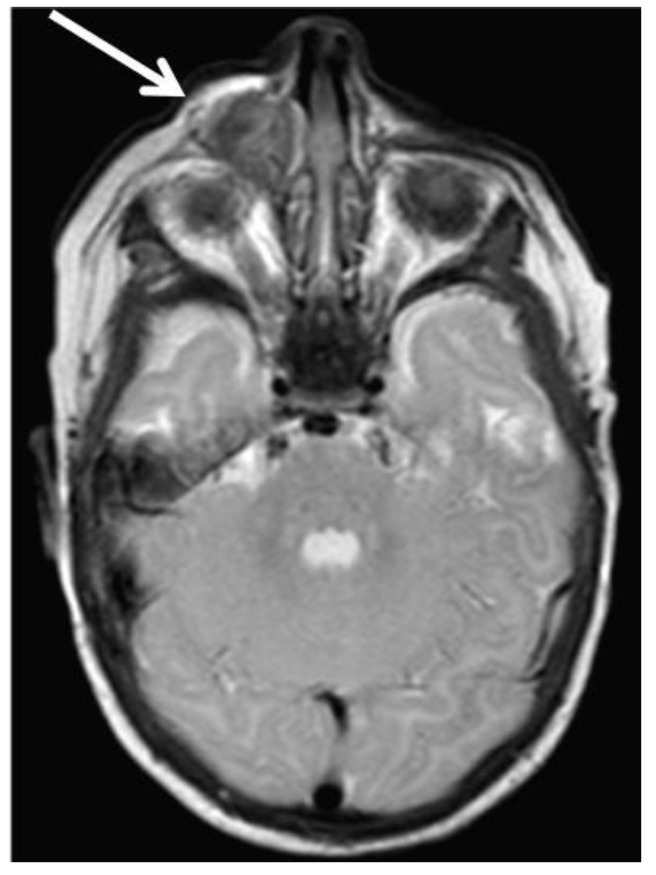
Patient 1: an axial T2-weighted MR scan shows well-defined pathological solid mass originating from the alveolar process of the right maxilla.
